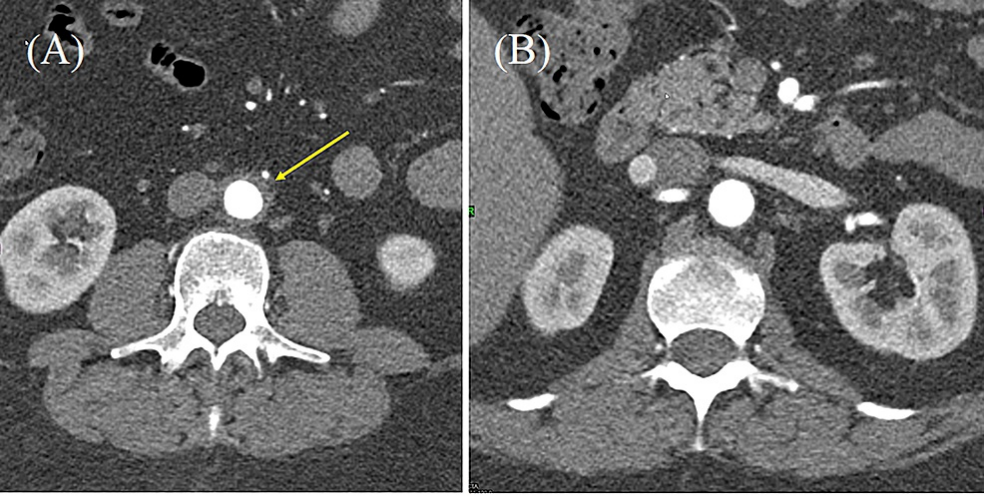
CT abdomen and pelvis with contrast (A) Mid-abdominal aorta with signs of wall thickening and adjacent inflammation (arrow); (B) level of upper abdominal aorta without signs of wall thickening and inflammation.
